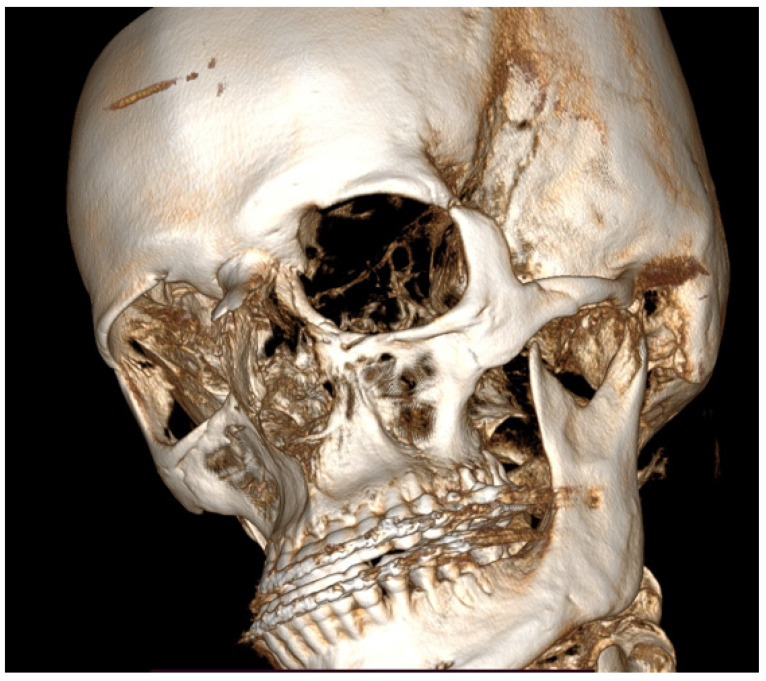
A CBCT-3D reconstruction with visible enlargement and overgrowth of the left mandibular condyle head. Also, a tendency for skeletal class III deformity is visible. In such cases of severe skeletal asymmetries, a necessity for orthognathic surgery procedures is quite important to improve facial symmetry, oval, and contour. The presented images are quite interesting because the unilateral condylar hyperplasia in this case slowly grew over the years and was not found before the first orthognathic procedure in the past; this was the main source of skeletal asymmetry relapse over the next years.
